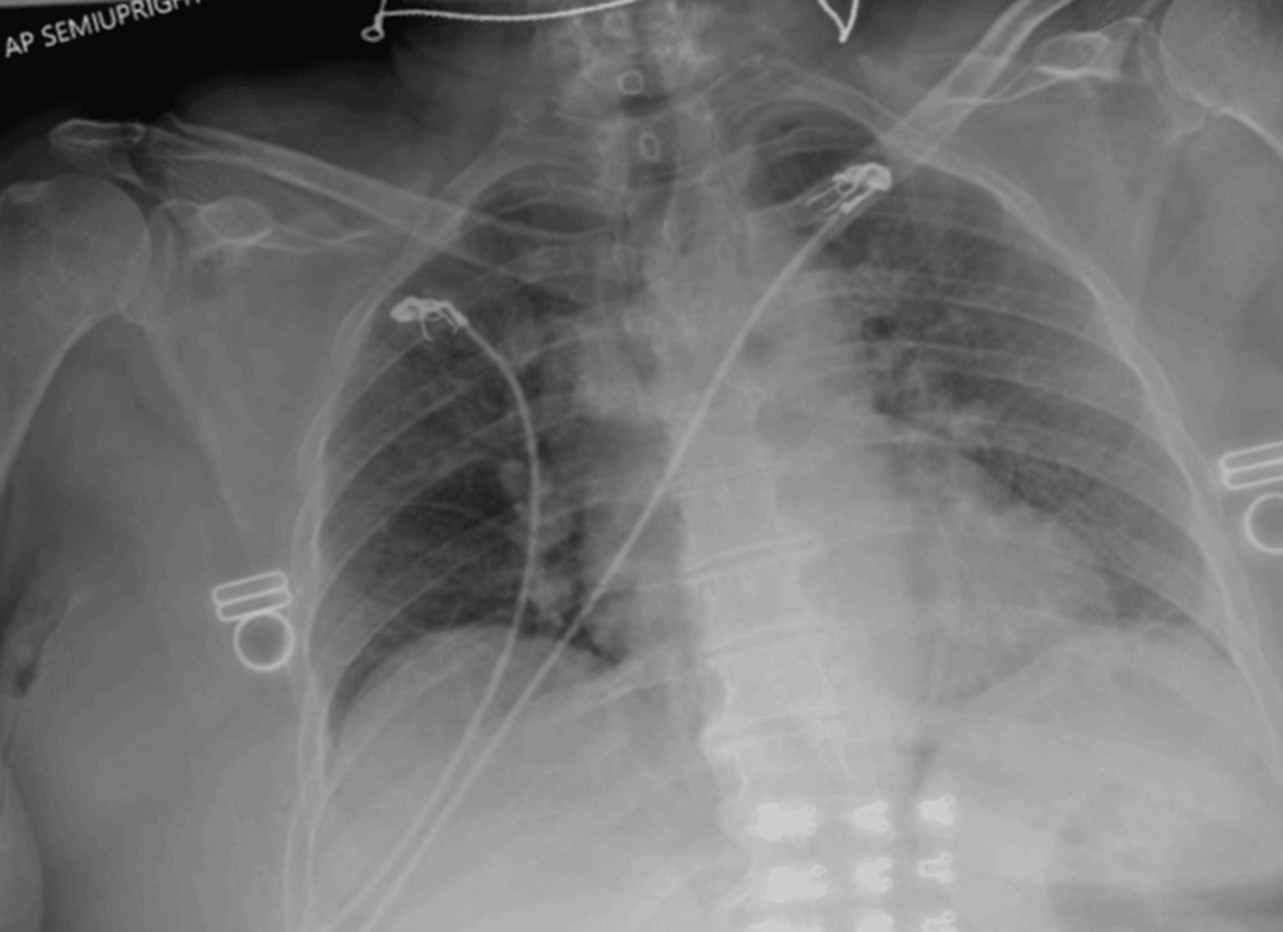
Anterior-posterior chest X-ray revealing mild diffuse interstitial prominence throughout the left greater than the right lung, potentially indicating atypical pneumonia.
